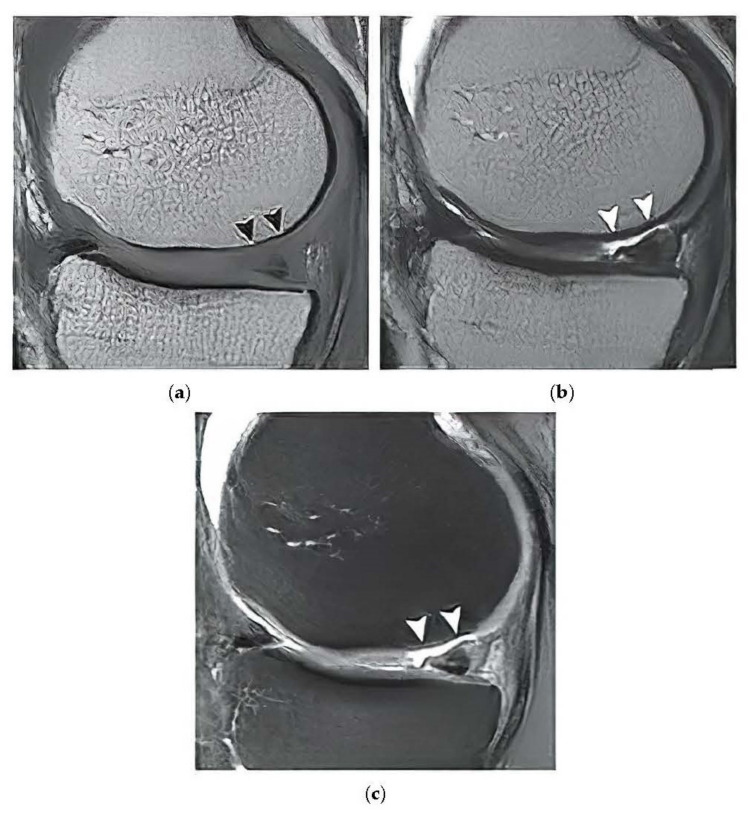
Example of sagittal 2D fast SE image, which was acquired based on the various MR sequences from the same knee’s area: (a) T1-weighted image shows a weak contrast between synovial fluid and cartilage surface. A significant limitation is not providing the information for a proper assessment of focal cartilage defect (arrows), (b,c): T2-weighted image (b) and weighted proton density (c), image provides a better contrast between the cartilage surface and synovial fluid, enabling the identification of a full cartilage defect (tip of arrow) in medial femoral condyle.
